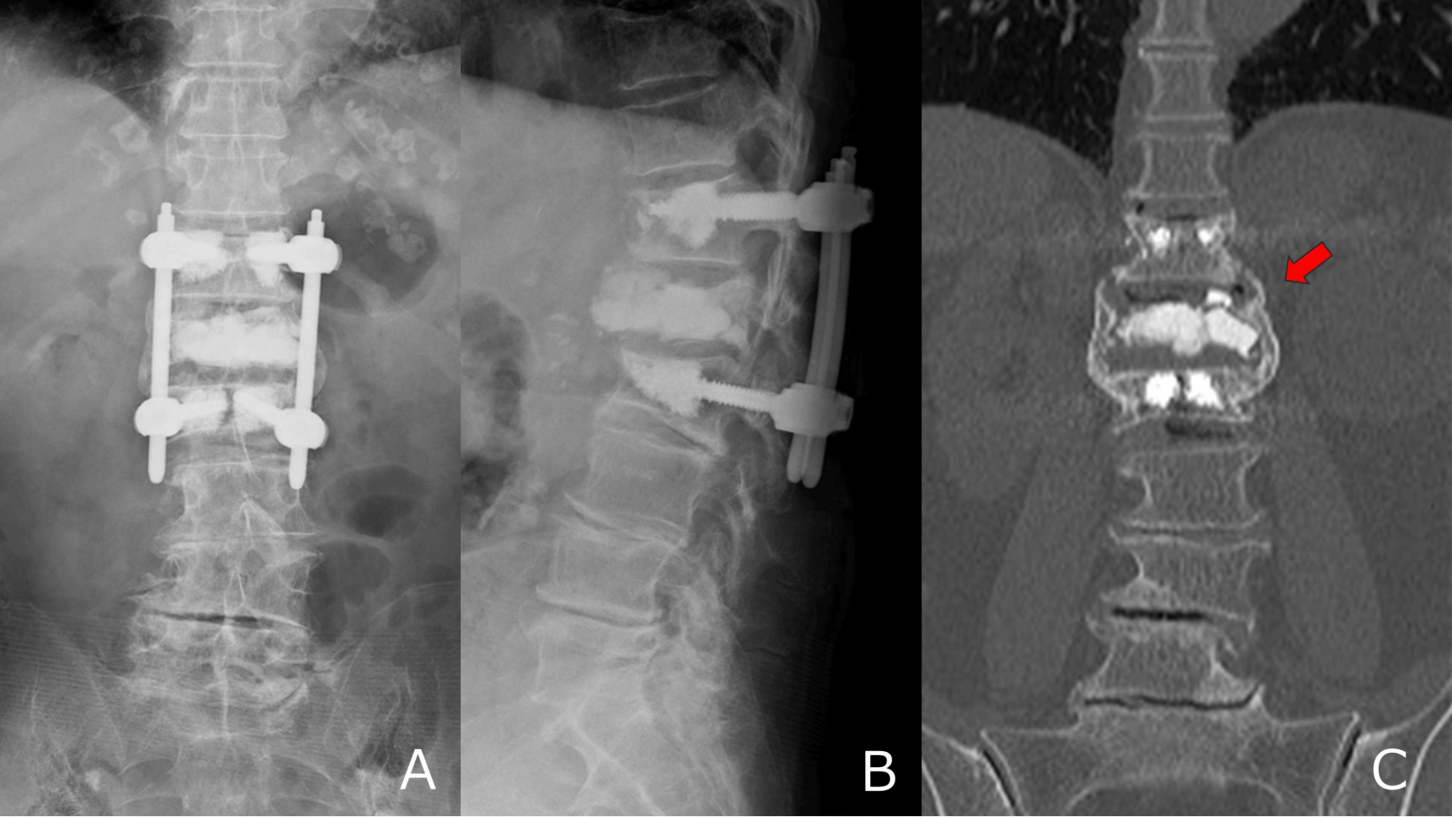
Postoperative imaging two years after surgery. (A–B) X-ray images showing no loss of correction. (C) Computed tomography (CT) image showing bony bridging on the lateral wall of the vertebral body (arrow).
